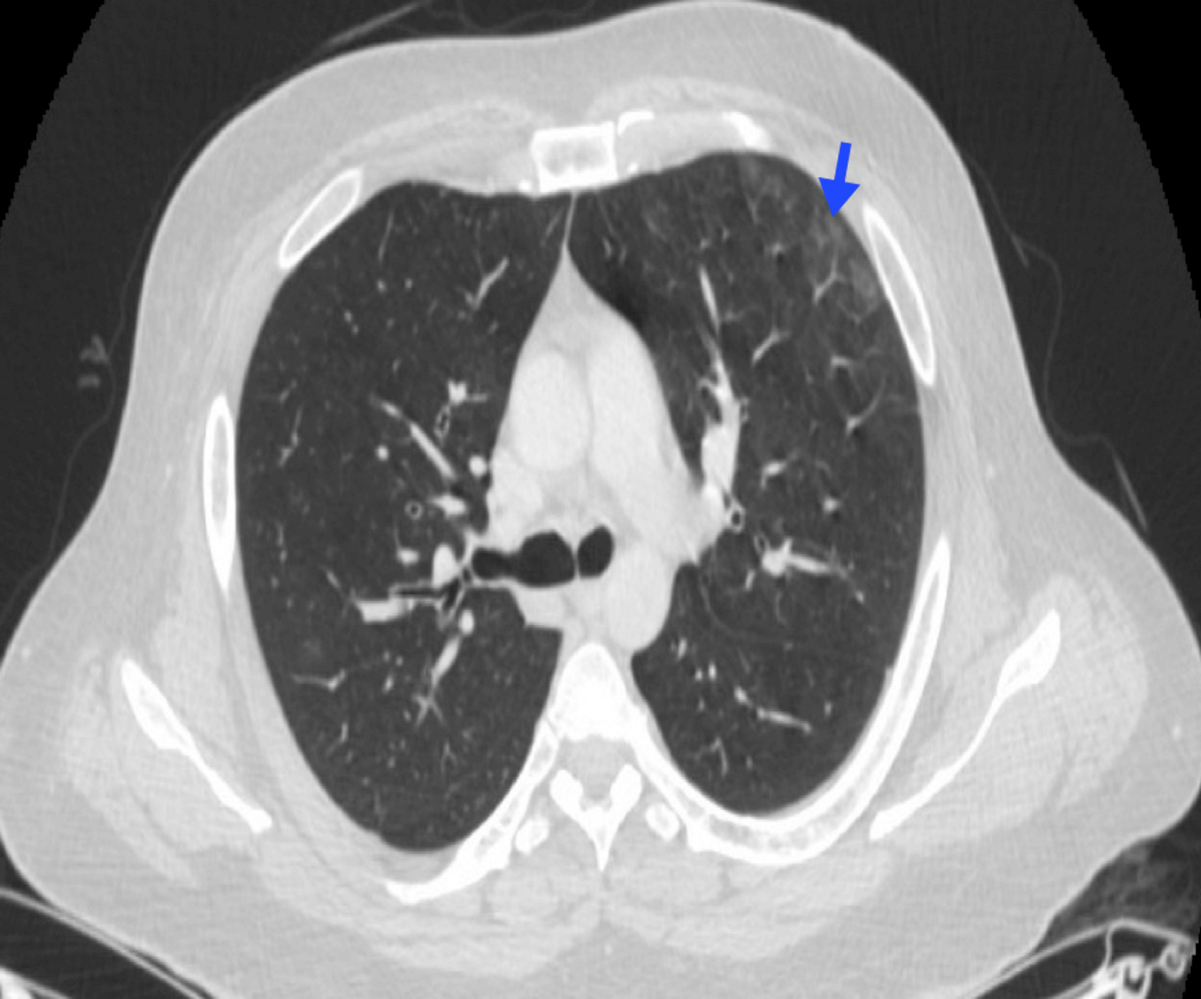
Leukemia lung involvement A 27-year-old male patient with the common phenotype of ALL. Chest CT reveals peripheral ground-glass opacities in the upper left lobe, along with central acinar micronodules of random distribution (blue arrow). Infectious causes were ruled out through BAL. ALL, acute lymphoblastic leukemia; BAL, bronchoalveolar lavage Image courtesy: Pulmonology Service of the Internal Medicine Department, University of Antioquia. Consent for open access publication was obtained from the patient.
